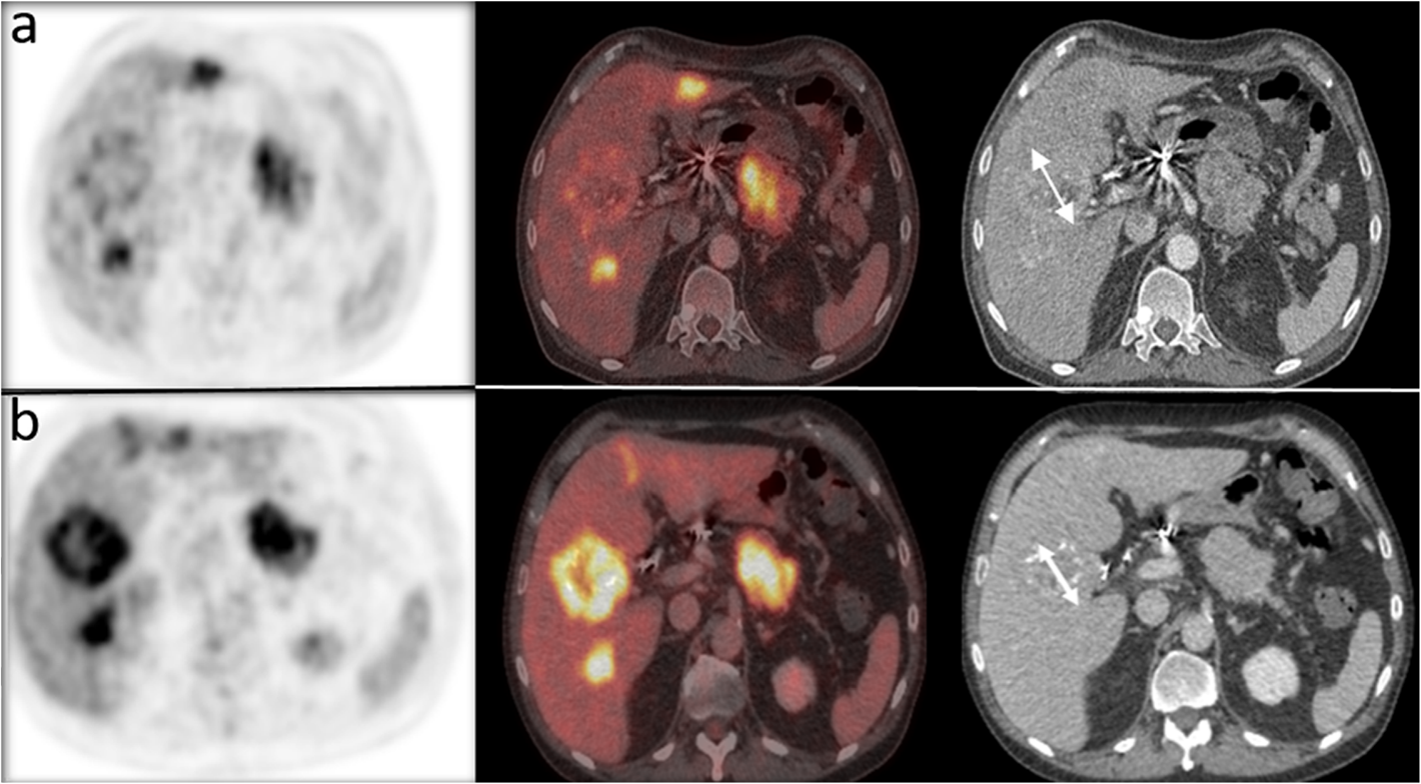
^18^F-FDG-PET/CT transverse images before PRRT (row a) compared to post-PRRT images (row b). Some liver lesions showed an increase in ^18^F-FDG uptake compared with baseline (left an middle images), although there was a morphologic therapy response on the CT images (right images) with new calcifications and a decrease in diameter (white arrow)
